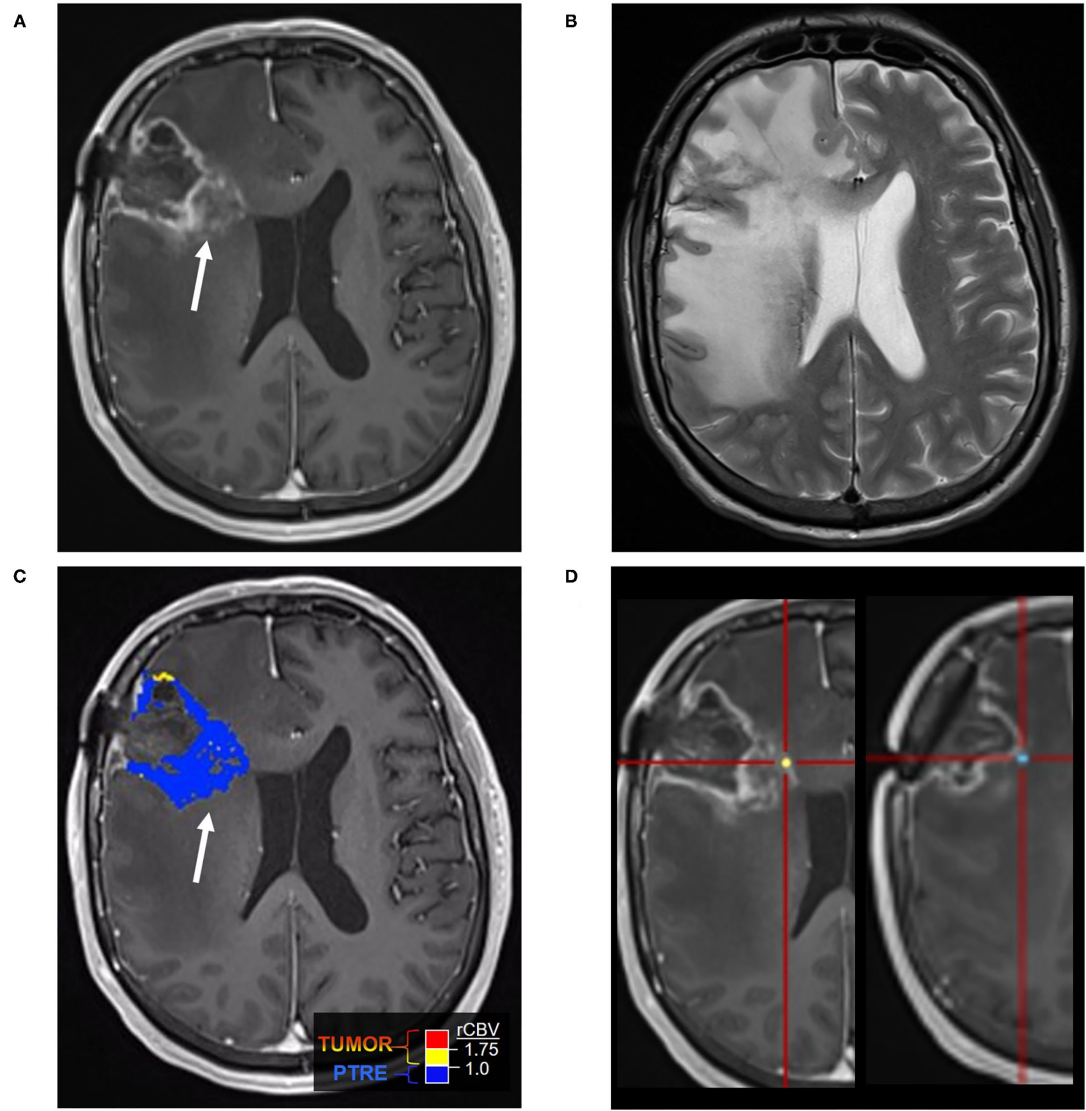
Posttreatment changes on Dynamic Susceptibility (DSC) MRI: A 58-year-old man with right frontal glioblastoma (GBM) status post resection and adjuvant radiation with concomitant temozolomide presented 6 months after resection with left facial droop and left-sided weakness. Initial CT and Contrast-enhanced MRI (CE-MRI) showed an increased mass effect and ill-defined enhancement around the resection cavity, indeterminant for recurrent tumor vs. posttreatment effects. (A) Axial postcontrast T1-weighted image demonstrates a linear enhancement around the right frontal resection cavity with more ill-defined nodular enhancement along the posteromedial margin (arrow). (B) Axial T2-weighted image shows T2 hyperintense signal and mass effect throughout the right frontal and parietal lobes. (C) DSC Fractional tumor burden (FTB) map derived from rCBV thresholds shows low FTB around the resection cavity favoring posttreatment changes (D) Stereotactic biopsies were performed and cross-registered retrospectively to areas of enhancement. Pathology demonstrated virtually entirely necrotic tissue with no viable tumor, concordant with the rCBV map.
